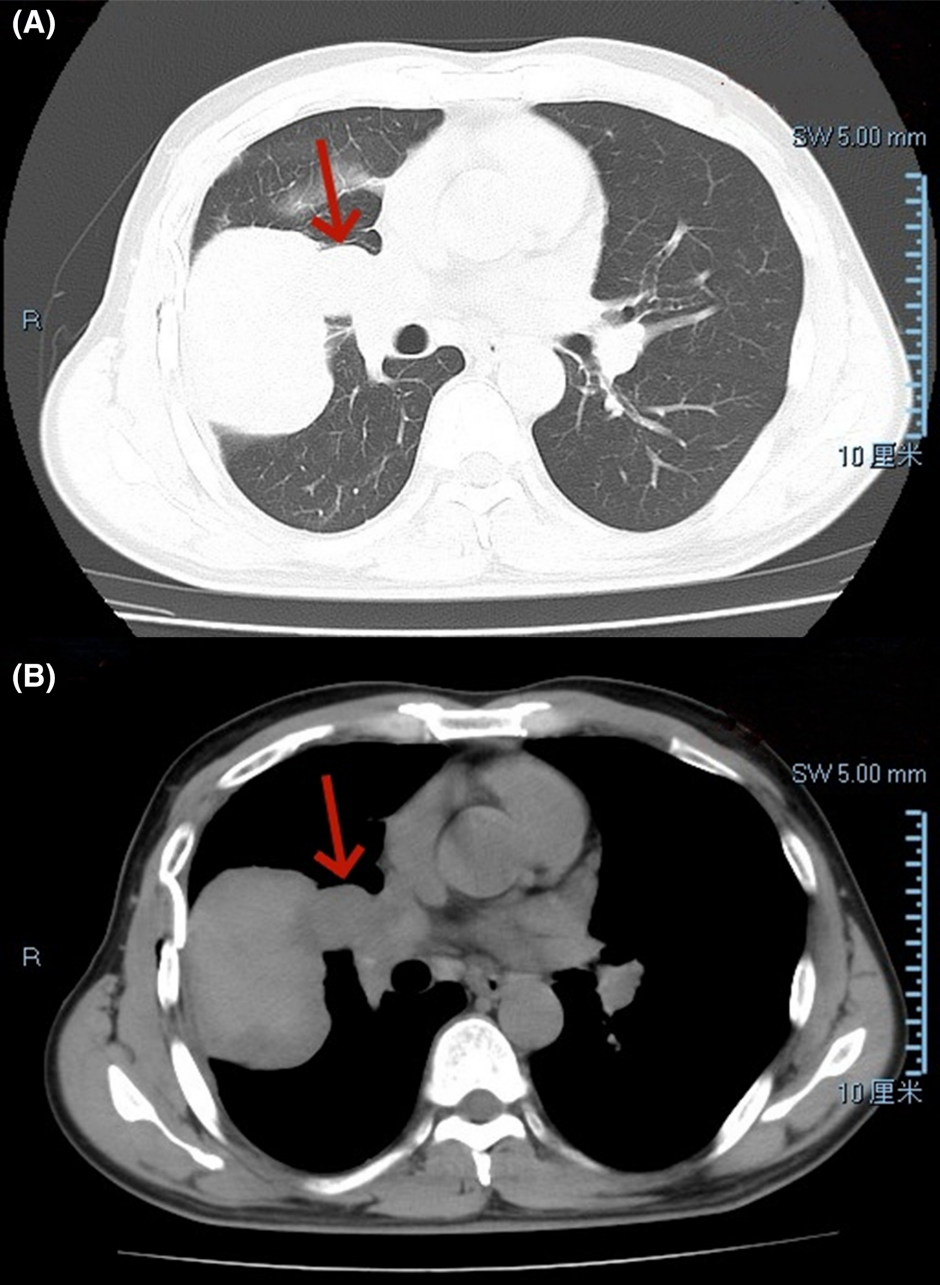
CT after drainage showed no significant change in the mass. (A) Shows the lung window manifestation on chest CT plain scan, and (B) Shows the mediastinal window manifestation.
